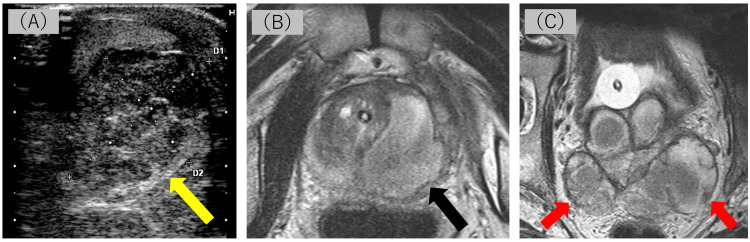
Ultrasonography and MRI at the time of hospital revisit. Scrotal ultrasonography shows a mosaic-like hypoechogenic area in the tail of the left epididymis (panel A). Pelvic T2-weighted MRI reveals fluid collection in the left lobe of the prostate and the left-dominant enlarged bilateral seminal vesicles (panels B and C). These images depict the simultaneous occurrence of abscesses of the prostate, bilateral seminal vesicles, and left epididymis. MRI, magnetic resonance imaging.
